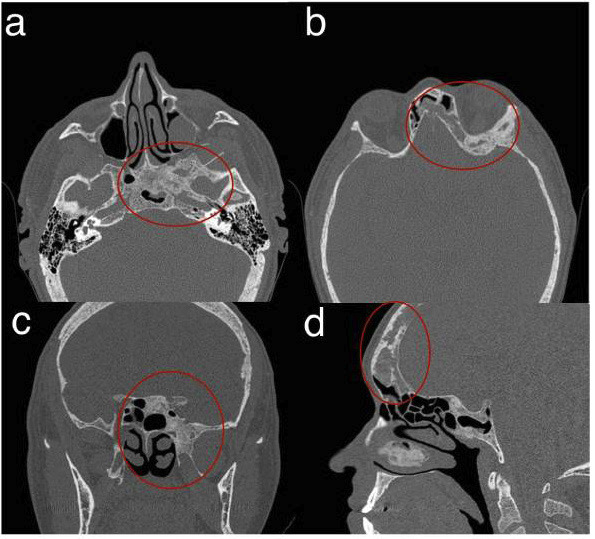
Computed Tomography Multi-slice: axial (a and b), coronal (c), and sagittal (d) scans showing hypodense images at the base of the skull and frontal region.
